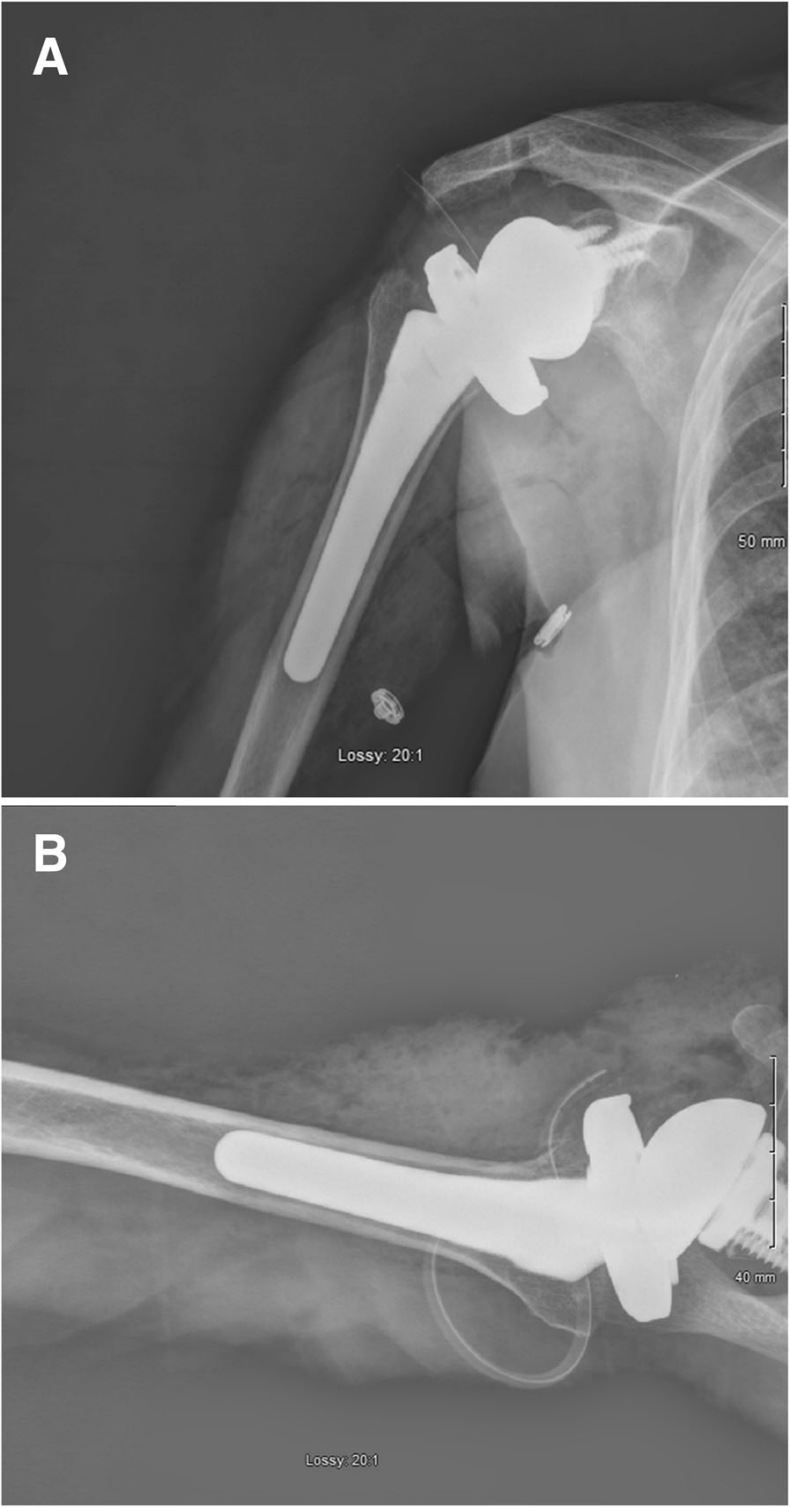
a-b: Post-operative radiographs demonstrating implant position and alignment
